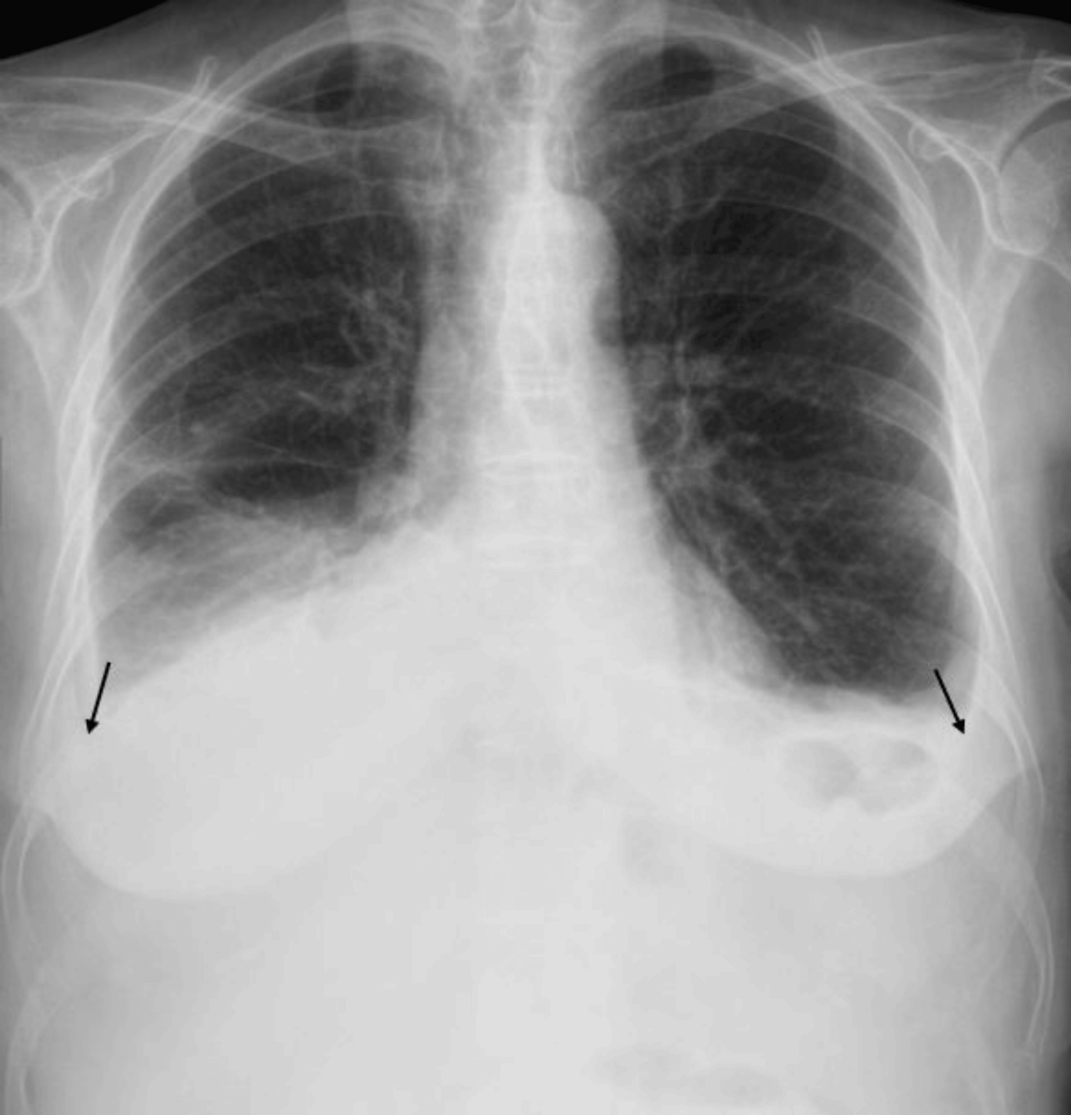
Chest radiography revealing bilateral pleural effusion (arrows).
